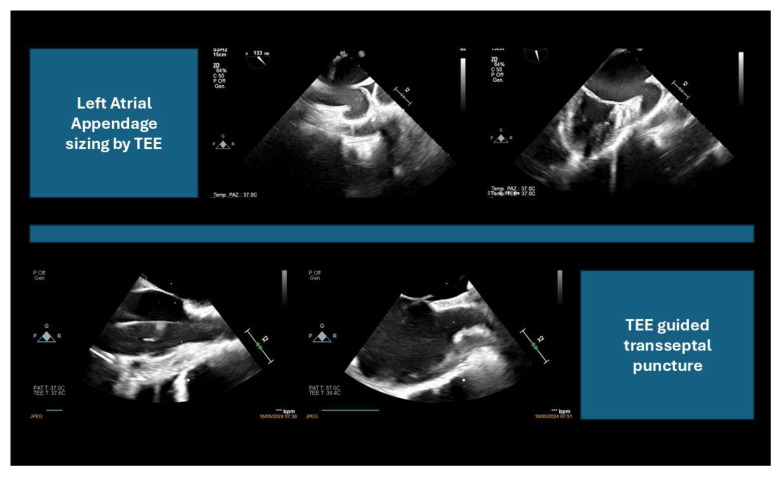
Left atrial appendage transesophageal echocardiography imaging: sizing and transseptal puncture.
